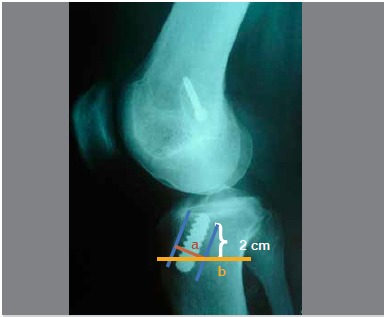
Profile radiography (P). A) The blue line follows the edges of the tunnel and at 2 cm below the medial joint line, perpendicular thereto, its diameter is represented by the line "a". At the same point the diameter of the bone is calculated, represented by line "b". The value obtained by the variable "a" is then divided by the constant .
